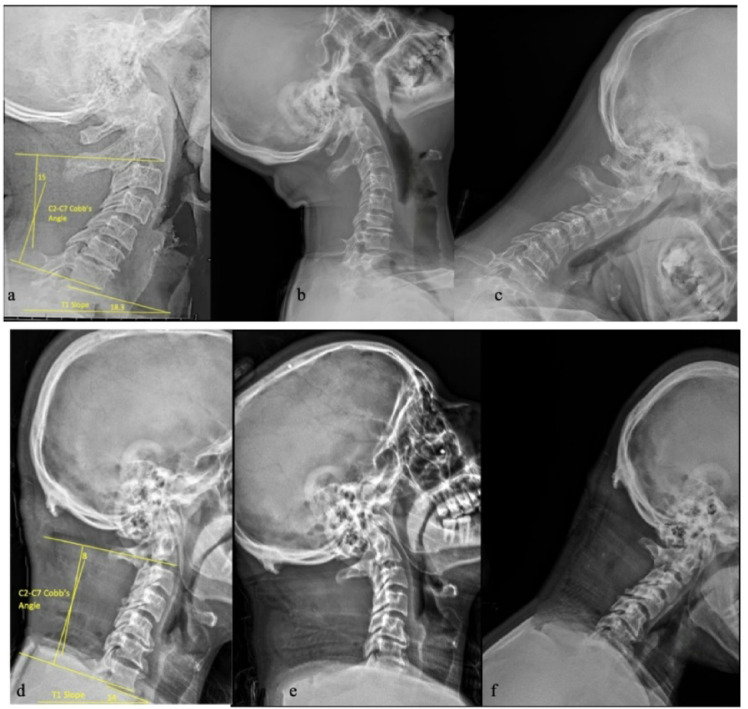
Postoperative lateral cervical spine X-ray at 2-year postoperative follow-up. Neutral standing lateral views (a, d) and flexion–extension views (b, c, e, f) following multilevel laminectomy show preserved motion without postoperative sagittal instability. C2–C7 lordosis and T1 slope were re-measured using the same method as baseline.
